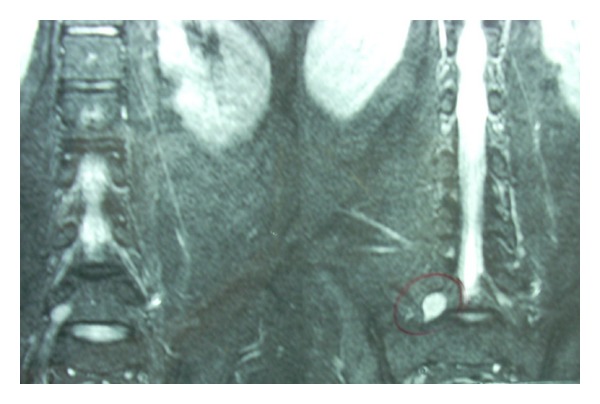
MRI T2W image (coronal) shows hyperintense nerve sheath tumour involving the right sided L5 nerve root.
